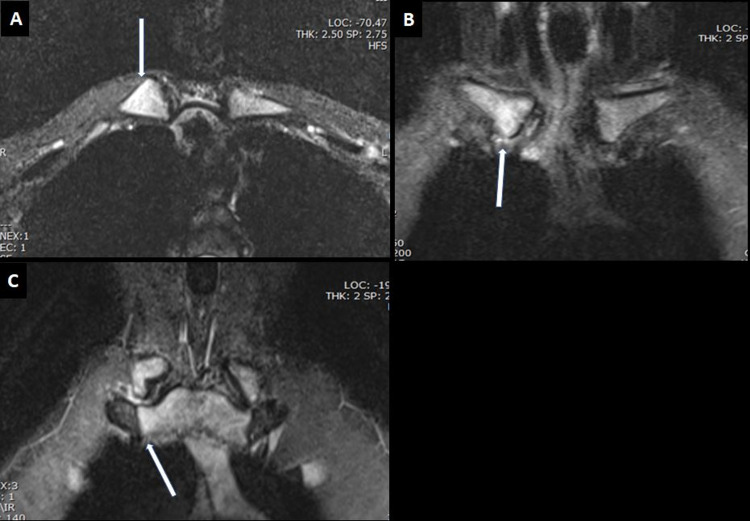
MRI of the sternoclavicular joints with contrast A. T1-weighted axial image showing abnormal marrow signal changes in the sternal end of the right clavicle (white arrow). B. T2-weighted coronal image demonstrating high abnormal signals in a similar area with mild right sternoclavicular effusion (white arrow). C. T1-weighted coronal image showing abnormal marrow signal changes in the right part of the manubrium sternum (white arrow) MRI: magnetic resonance imaging
